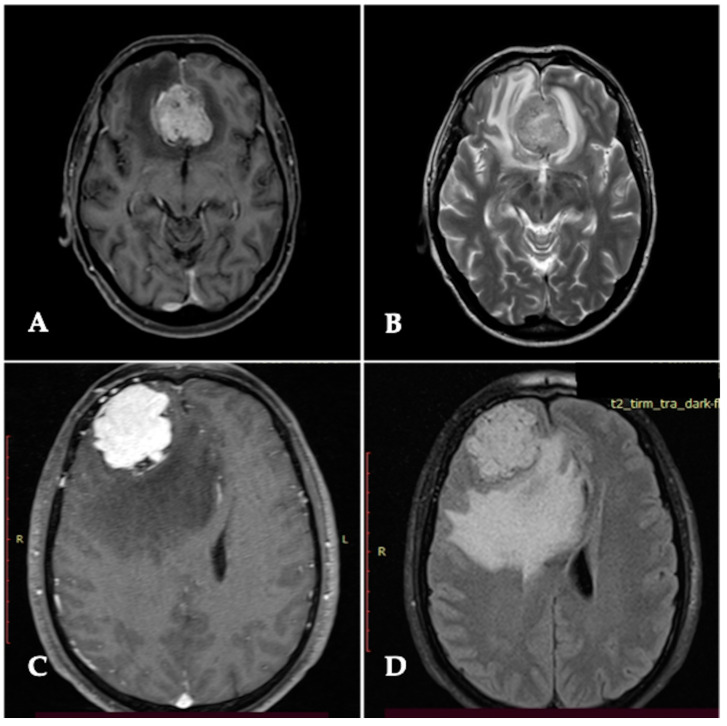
T2-weighted and FLAIR MRI demonstrating the degree of peritumoral edema between a skull-base meningioma (A,B) and a convexity meningioma (C,D), respectively. Some authors correlate it to tumor grade, consistent with lower grade meningioma in skull-base located meningioma and higher grade in convexity located meningioma.
